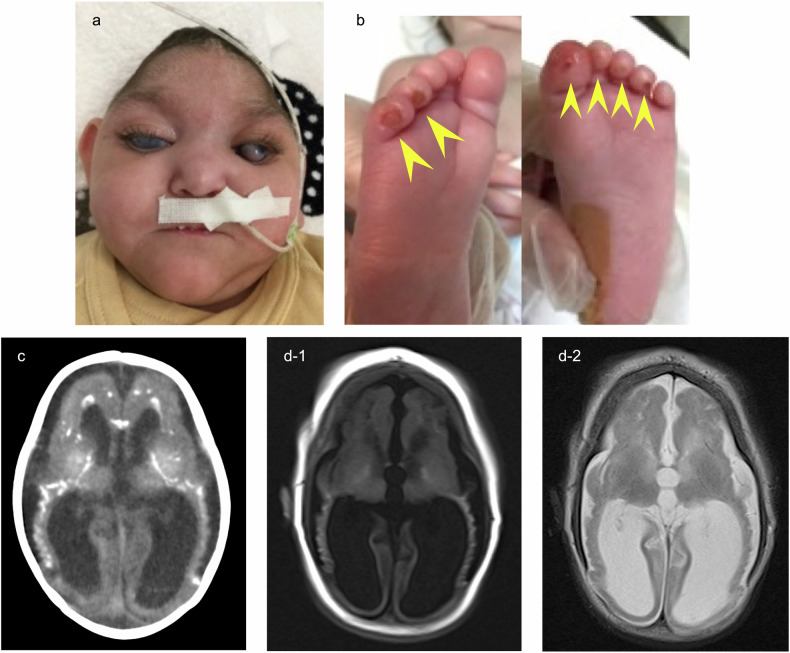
Patient details. a Patient at 12 months of age with microcephaly and bilateral cataracts. b Chilblain-like lesions (yellow arrowheads) at 2 months of age. c Brain CT at 1 month of age showing calcification of the basal ganglia and white matter. d Brain MRI (d-1 T1-weighted, d-2 T2-weighted) at 4 months of age revealed cerebral atrophy and polymicrogyria.
